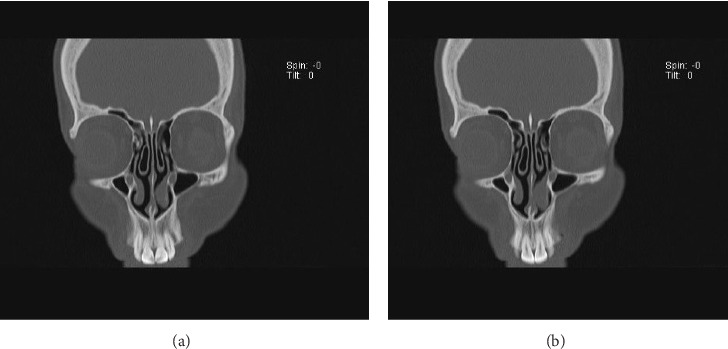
A 47-year-old female presented with progressive nasal obstruction and was found to have a myxoma arising within the inferior nasal concha. The preoperative coronal section of the PNS CT scan demonstrates an expansile mass.
